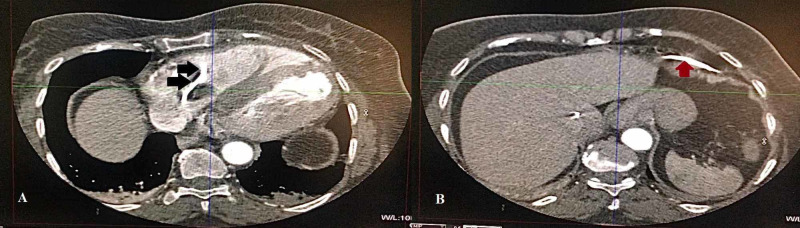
(A, B) An axial plane of CT showing the temporary pacing wire (black and red arrows) going through the right ventricle and into the abdominal cavity.
